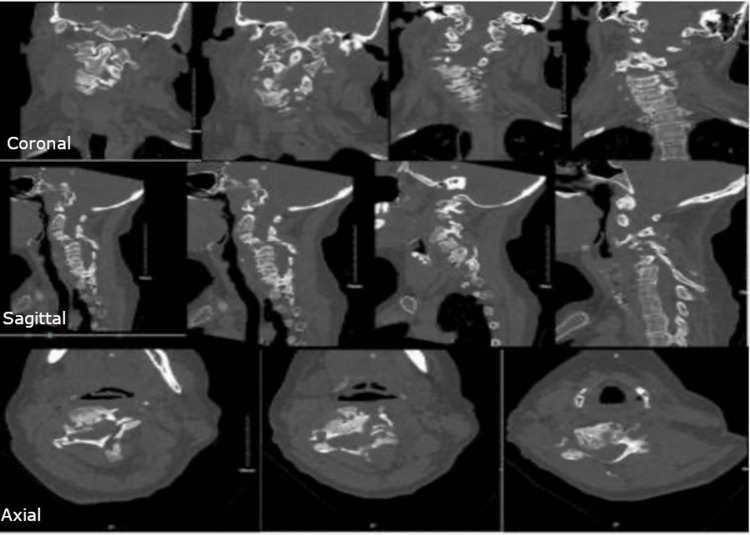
Preoperative Cervical CT Preoperative cervical CT images (sagittal, coronal, axial) demonstrating left-sided facet dislocation at C4-5, autofusion across the C3-4 disc space and right facet joint, asymmetric pedicle bone loss, and rigid cervical kyphoscoliosis.
